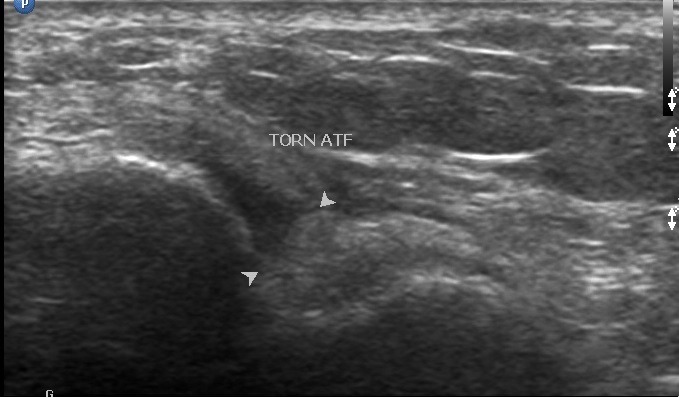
anterior talofibular ligament tear, 21 year old female patient with history of an ankle sprain. Ultrasound of the lateral compartment demonstrates a discontinuous ligament (arrow heads) with loss of attachment to the talus replaced by echogenic tissue. (ATF=Anterior talofibular ligament)
